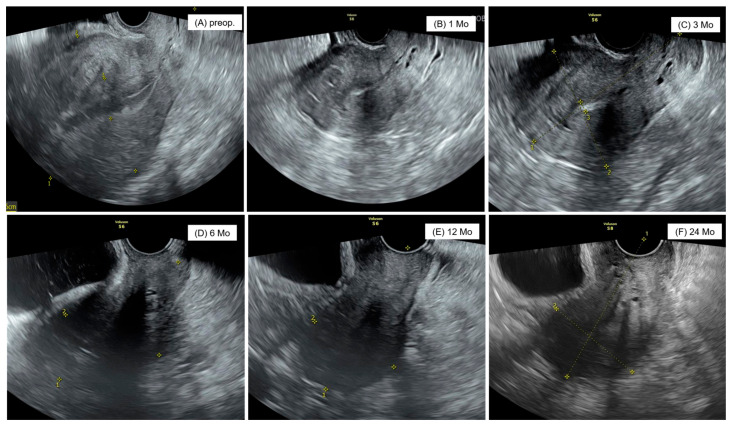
Postoperative ultrasound image of uterus in patient undergone with single-site laparoscopic fundusectomy. (A) preoperative, (B) at 1 Mo, (C) at 3 Mo, (D) at 6 Mo, (E) at 12 Mo, and (F) at 24 Mo.
